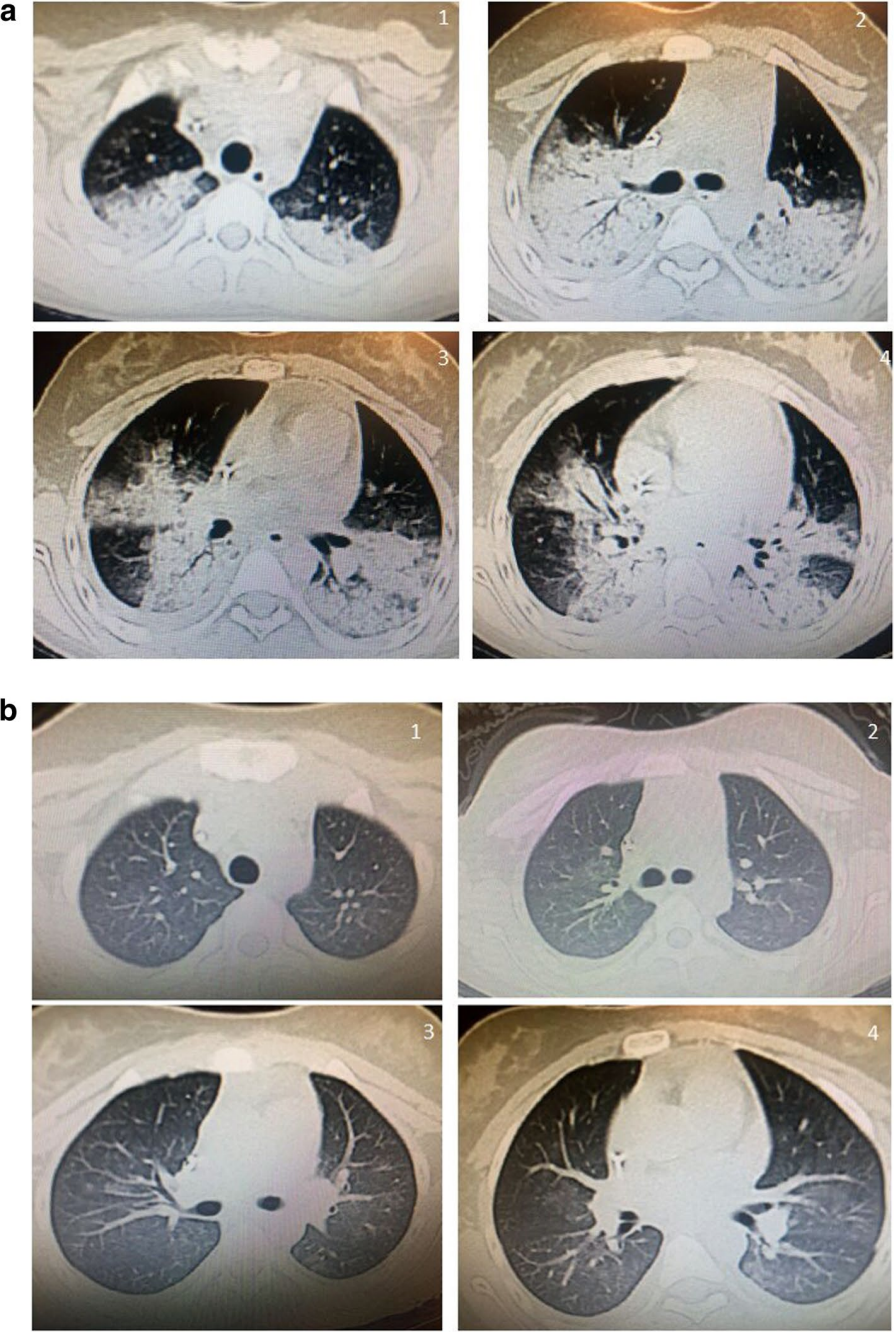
a Case 1 initial serial CT cuts showing the large bilateral pulmonary predominantly posterior diffuse areas of ground-glass opacities and consolidations with related accentuated vascularity and septal thickening (CO-RADS 5). b Follow-up case 1 serial CT cuts showing significant regressive course with near total resolution but still seen bilateral diffuse mosaic attenuation of ground glass pattern
